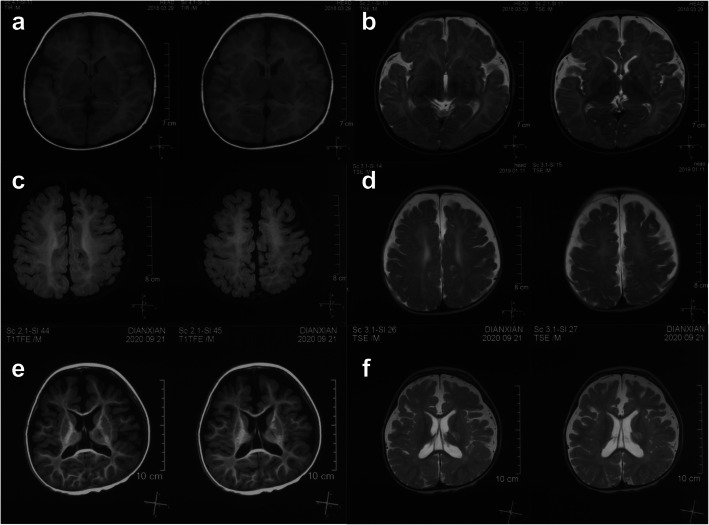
Brain MRI of Patient 1 at age of 5 months (a, b), 1 year and 3 months (c, d) and 2 years and 11 months (e, f). Agenesis of corpus callosum, bilateral frontal atrophy, and white matter hypomyelination were shown
